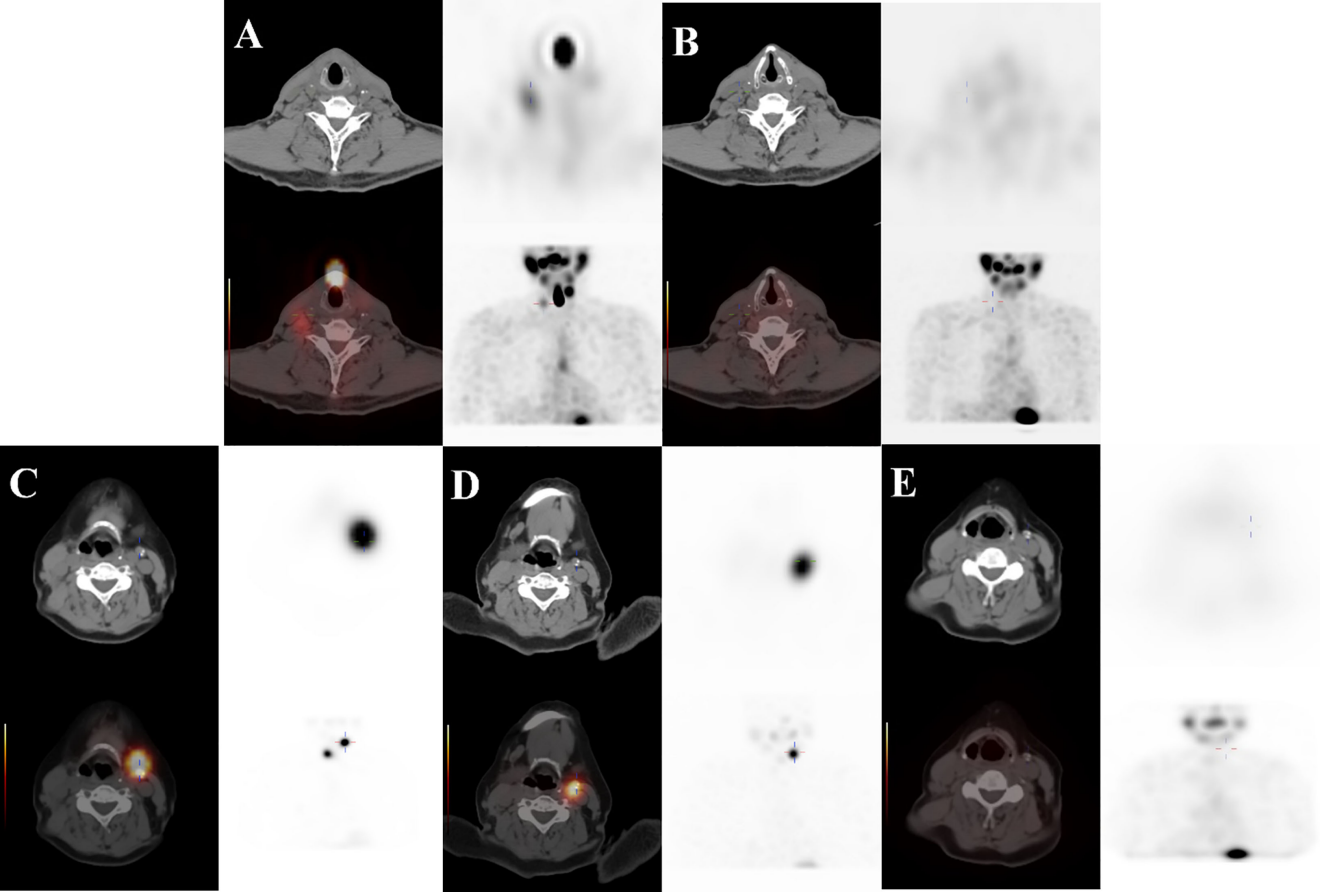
① A 71-year-old male DTC patient with ^131^I+ LNM on the right neck at Level III. (A, B) The first PTS was positive (TSH: 70.411 mIU/l, Tg: 19.2 μg/l, TgAb: <10 IU/ml), and the second PTS was negative (TSH: 82.545 mIU/l, Tg: 1.97 μg/l, TgAb: <10 IU/ml). She was classified as ER at the end of follow-up (TSH: 0.891 mIU/l, Tg: 0.04 μg/l, TgAb: <10 IU/ml, negative ultrasound features). ② A 67-year-old female DTC patient with ^131^I+ LNM on the left neck at Level II. (C–E) The patient’s first two PTSs were positive, and the third PTS was negative (TSH: 57.084 mIU/l, Tg: 39.58 μg/l, TgAb: <10 IU/ml→TSH: 88.895 mIU/l, Tg: 8.68 μg/l, TgAb: <10 IU/ml→TSH: 74.001 mIU/l, Tg: 3.79 μg/l, TgAb: <10 IU/ml), and she was classified as IDR at the end of follow-up (TSH: 0.019 mIU/l, Tg: 0.04 μg/l, TgAb: <10 IU/ml, calcification on ultrasound).
